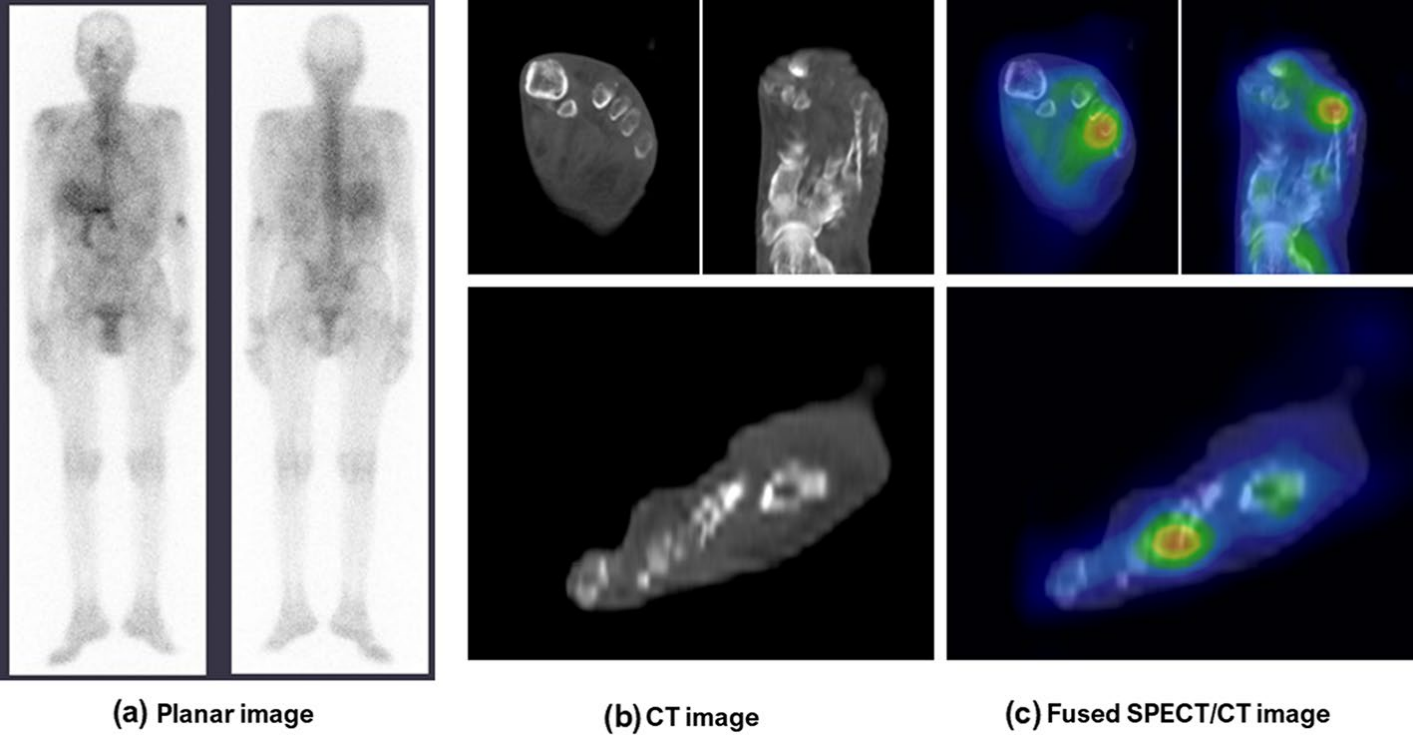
These images represent a 68-year-old man who developed a fever and increased inflammatory markers after treatment for leg trauma, with dyslipidemia, stable angina, and atherosclerosis obliterans as comorbidities. a shows Ga-scintigraphy, whole-body, planar images indicating a defect in the left toes with no clear signs of accumulation. b shows CT images indicating a resection of the left toes and cellulitis near the left 4th distal phalanx with irregular bone destruction, and increased attenuation lesions in the subcutaneous soft tissue. c shows fused SPECT/CT images indicating distinct accumulation in the left 4th distal metatarsal and proximal phalanx with low LBR (5.40), SUVmax (3.25), and TLU (35.02). Recovery from fever and inflammation was smooth, not requiring surgical treatment, but localized pain remained for a few months. The clinical diagnoses of CE and LLOM were established by the primary physicians according to the clinical progress and outcomes. This patient had no MAE within the 3-year observation period
